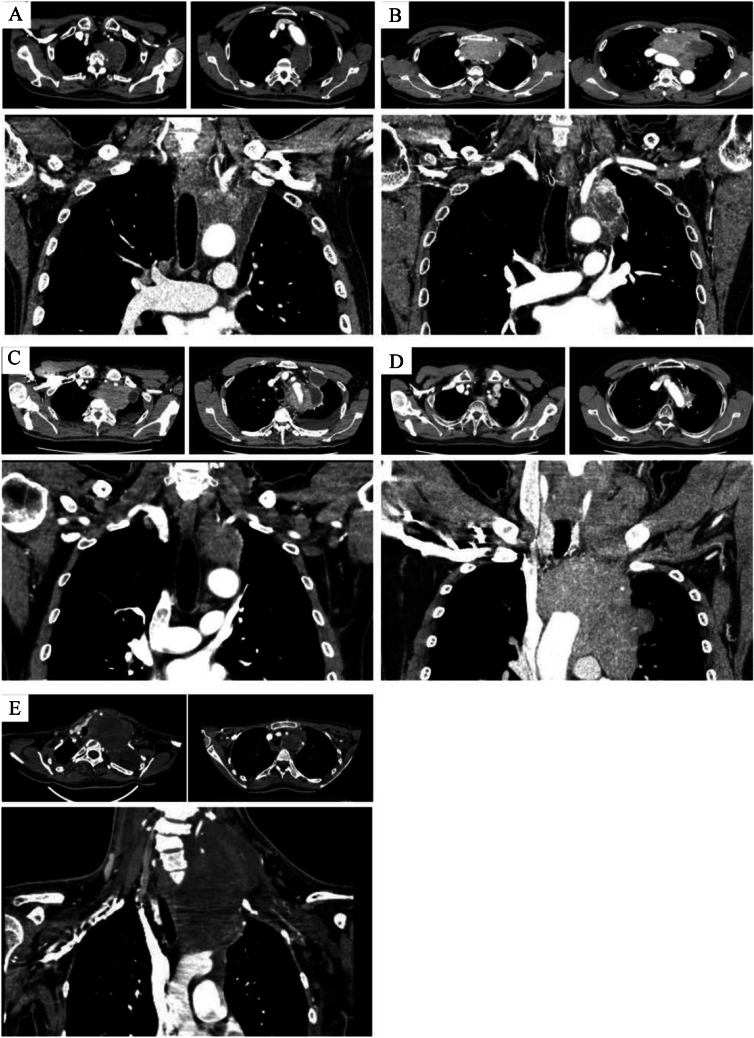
A. Computed tomography (CT) images showing a tumor with suspected invasion of the lung, aorta, vertebra and subclavian artery (Case 1). B. CT images showing a tumor with suspected invasion of the lung, hilar, and aorta (Case 2). C. CT images showing a tumor with suspected invasion of the lung, aorta, subclavian artery, and phrenic nerve (Case 3). D. CT images showing a tumor with suspected invasion of the lung, aorta, and subclavian artery, with possible extension to the neck (Case 4). E. CT images showing a tumor with suspected invasion of the left subclavian artery and subclavian vein, with possible extension to the neck (Case 5). *SCA* subclavian artery, *SCV* subclavian vein
